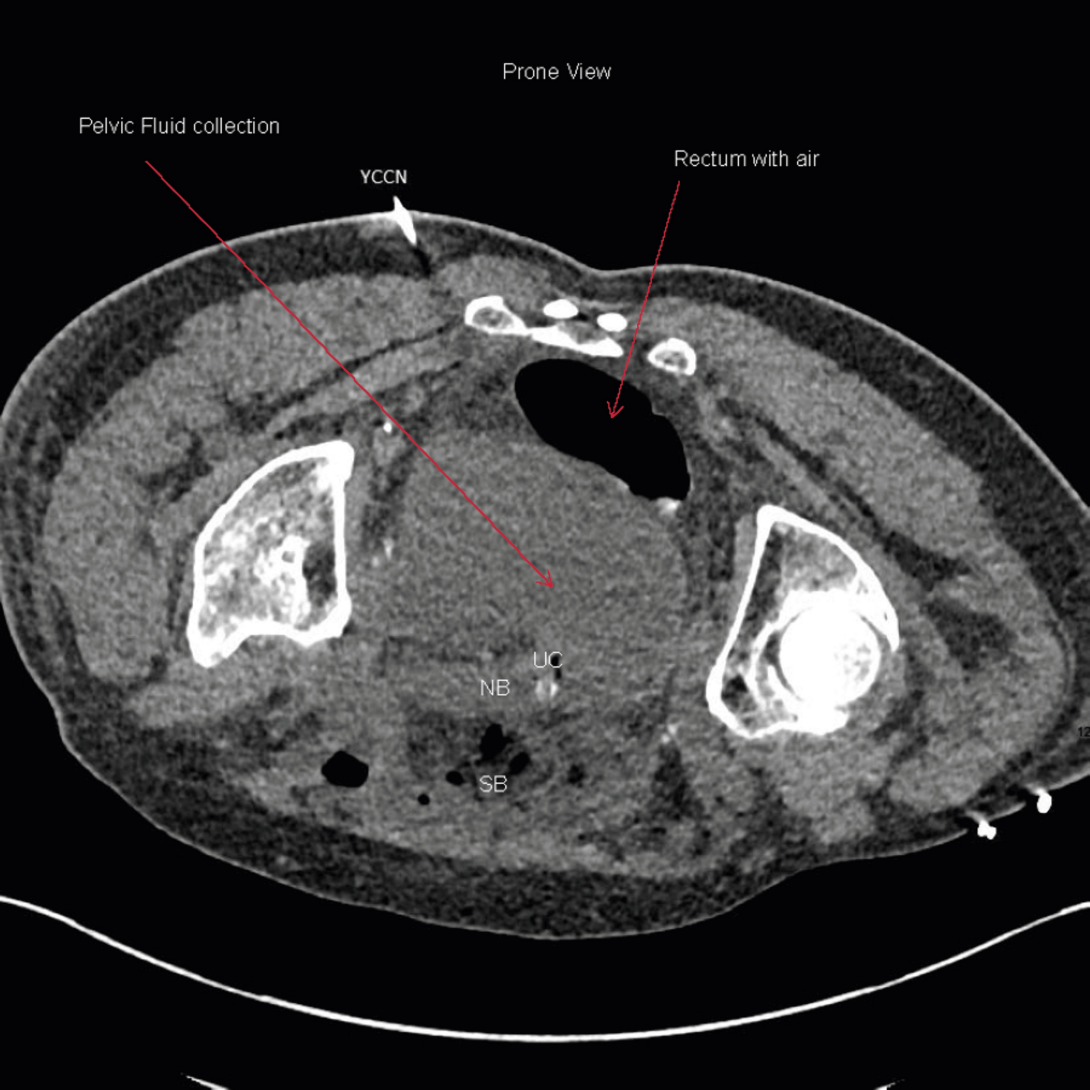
Axial prone CT view showing cutaneous entry path of CT-guided insertion of Yueh Centesis Catheter Needle (YCCN) (Cook Medical, Limerick, Ireland) to insert the pigtail drain into the pelvis
